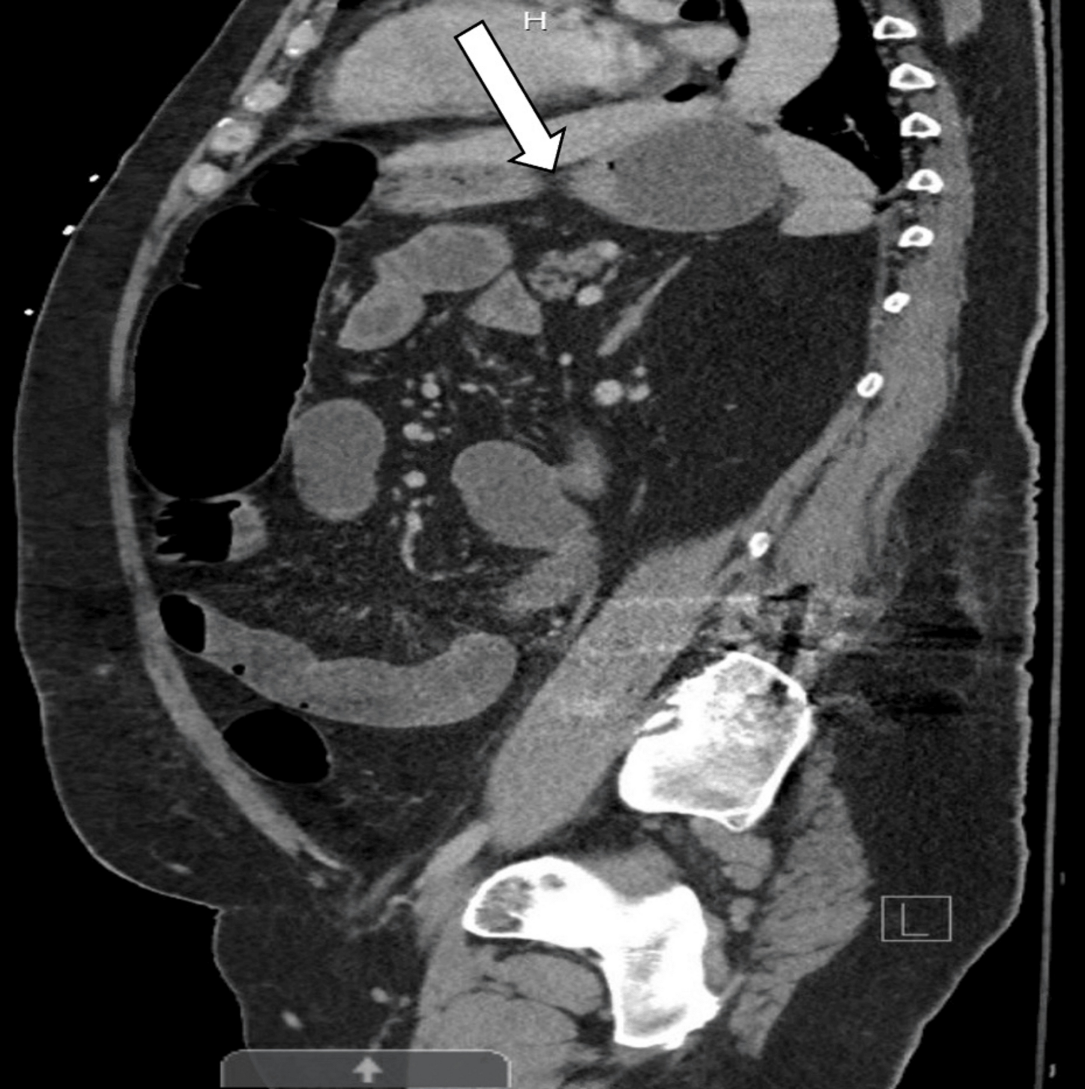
A CT scan of the abdomen and pelvis showed that the transition point was in the mid-descending colon (arrow).
